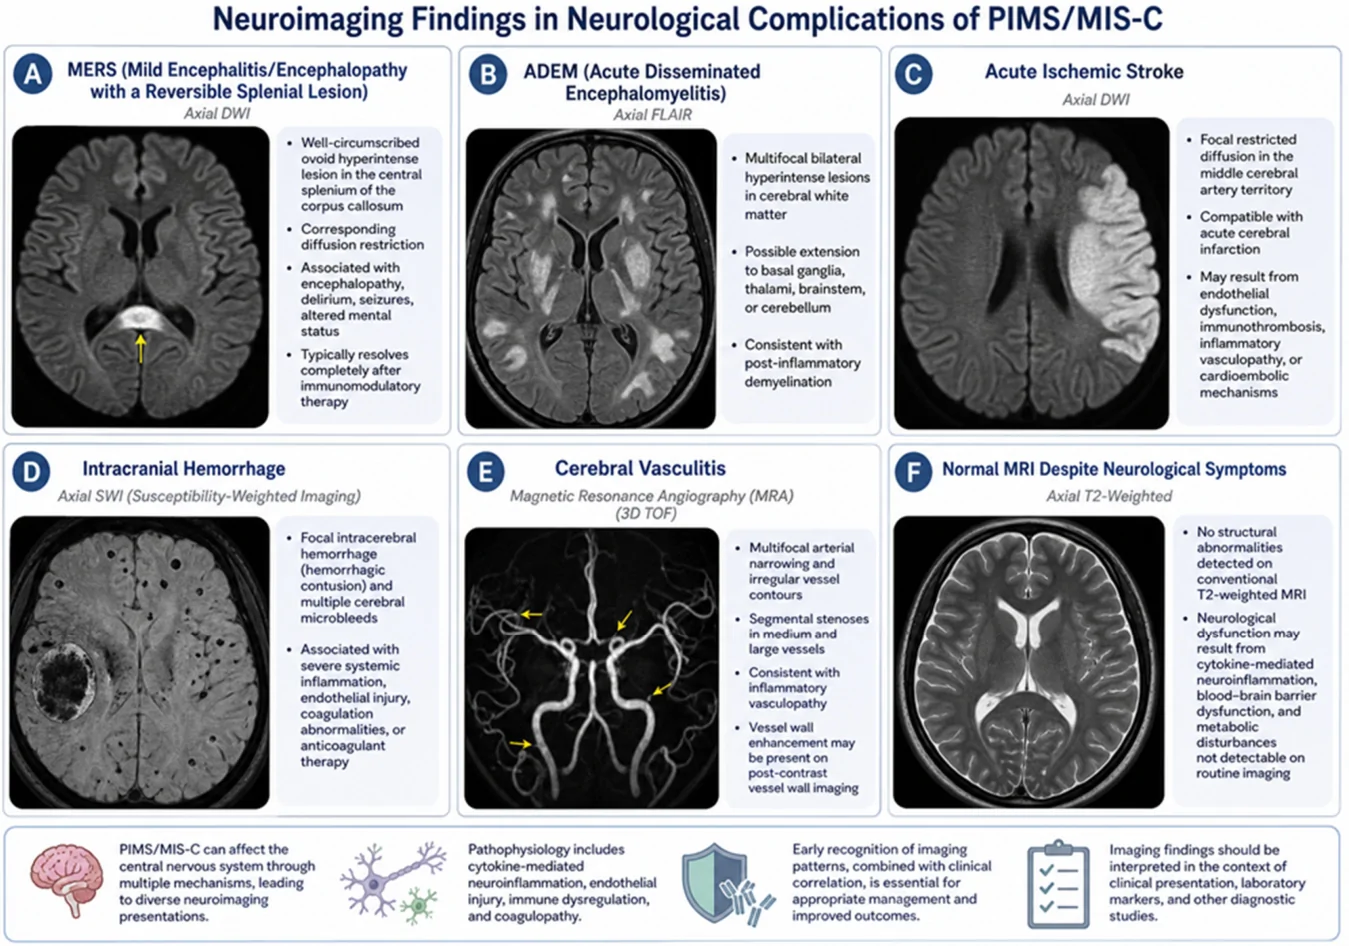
Representative magnetic resonance imaging findings in children with neurological involvement in PIMS/MIS-C.
